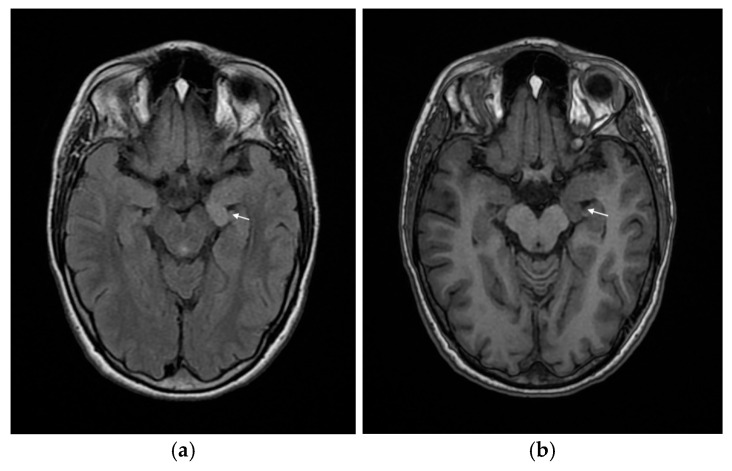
MRI of the CNS of the patient, showing neuronal heterotopy of the left hippocampus: (a) axial plane, FLAIR (small white arrow points the finding) (b) axial plane, T1 (small white arrow points the finding).
